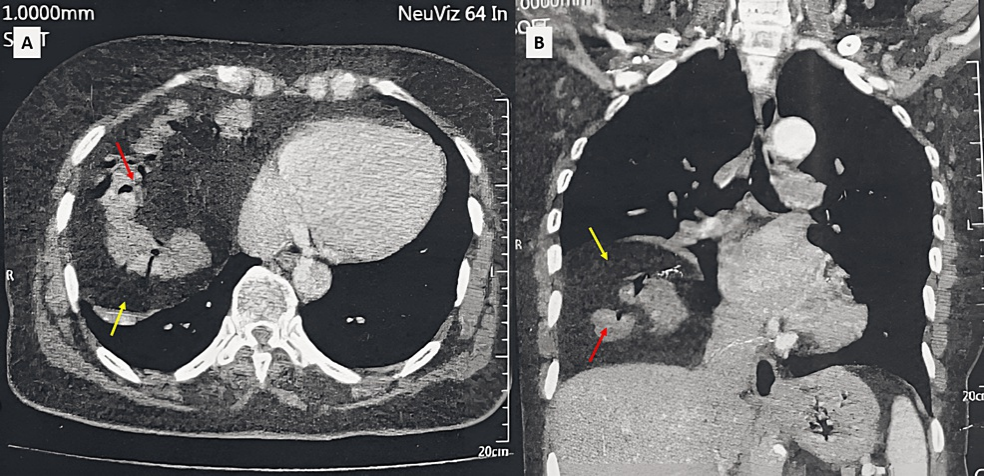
Axial (A) and coronal (B) sections of chest CT scan showing an ascent of a portion of the transverse colon (red arrow) and omentum (yellow arrow) through an anterior retrosternal defect.
